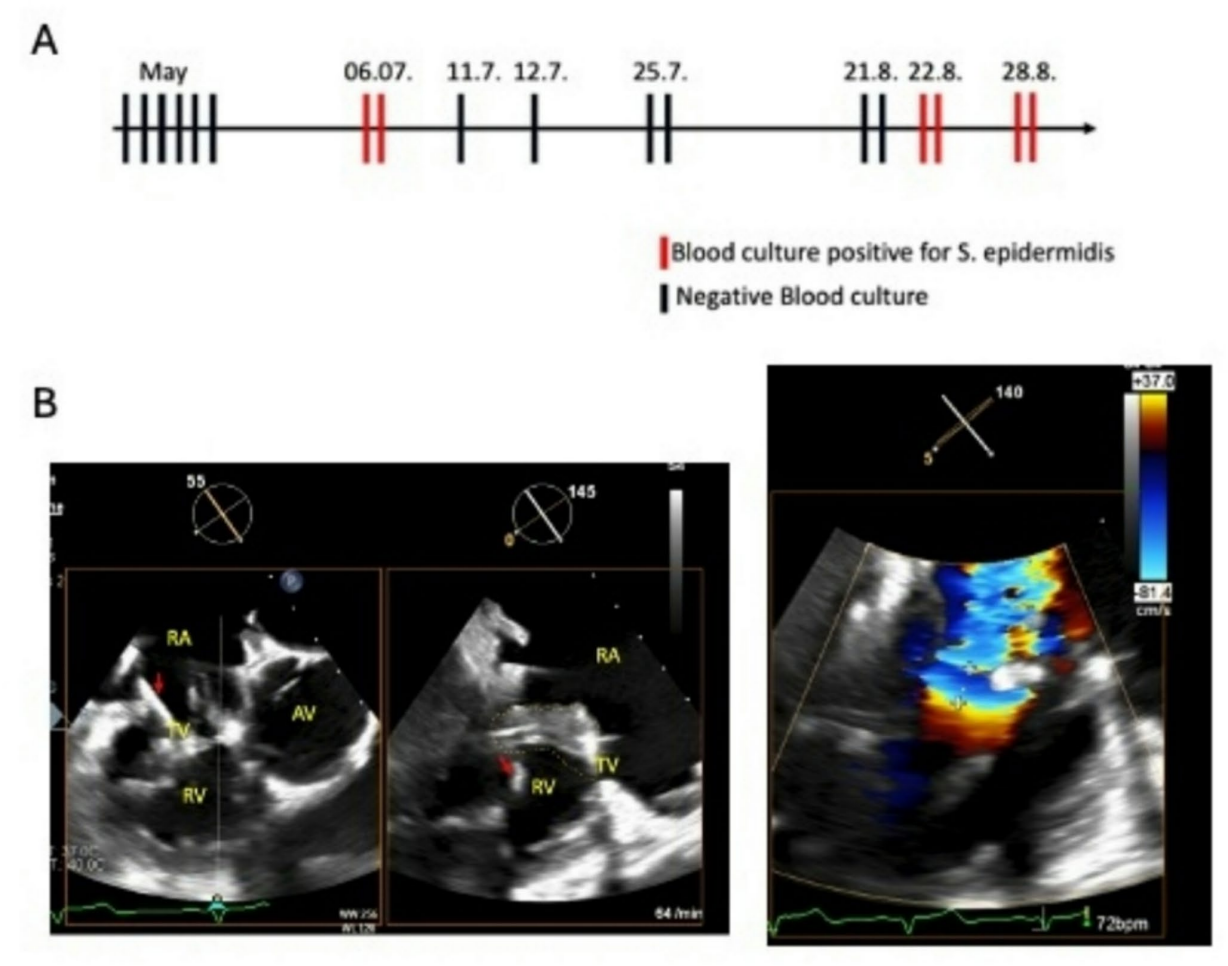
A) Blood Culture results until hospital admission. Each line represents one pair of aerobic and anerobic cultures. B) Transesophageal echocardiography indicating endocarditic vegetations along the tricuspid valve and CRT leads (right, red arrow) followed by tricuspid regurgitation (right). RA: right atrium, RV: right ventricle, AV: aortic valve, TV: tricuspid valve
